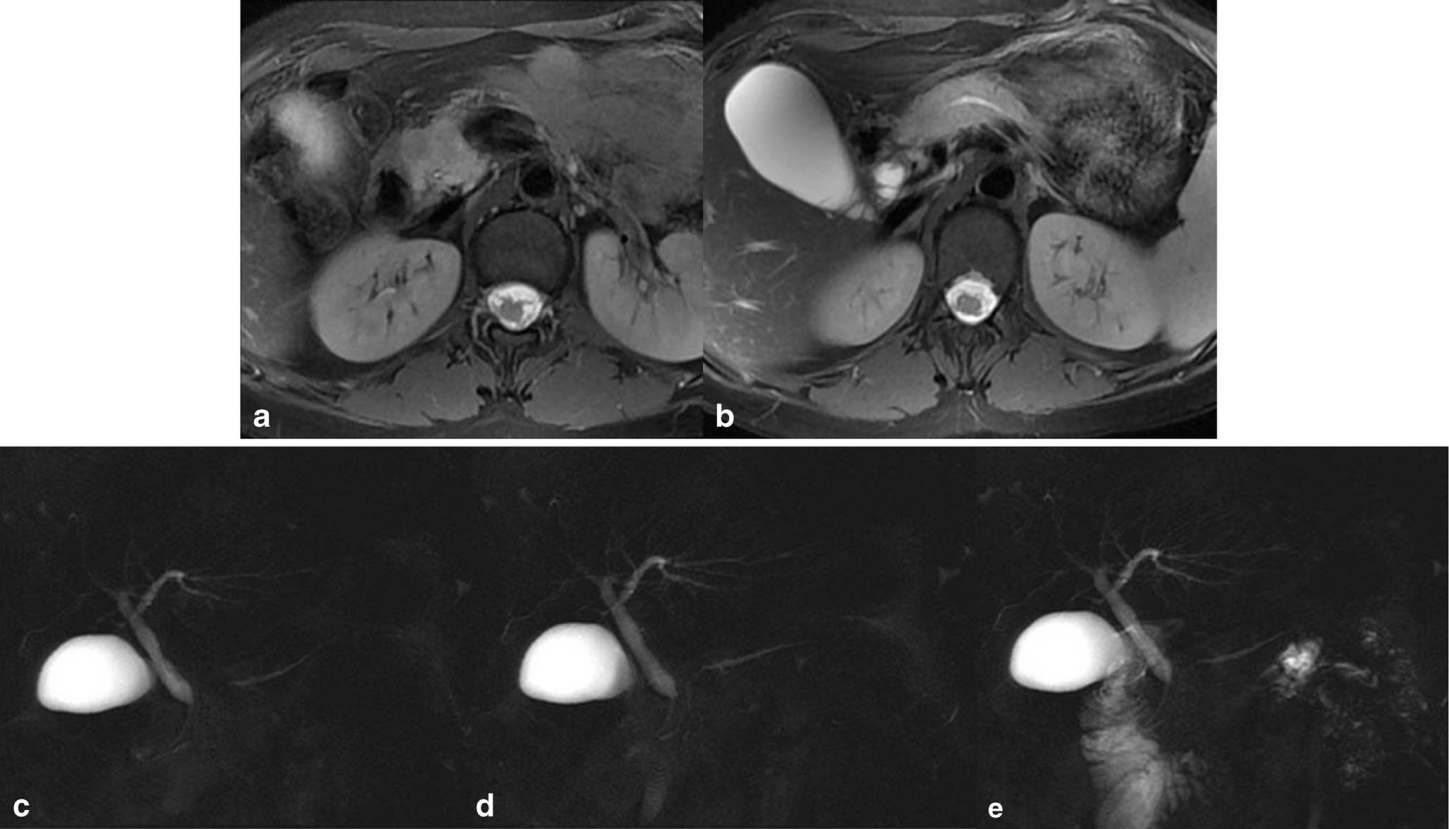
a–e Autoimmune pancreatitis. Axial T2-weighted images (a, b) show loss of the normal pancreatic lobulations at the level of the head and focal obliteration of the pancreatic duct, better demonstrated on conventional MRCP (c). After secretin administration (d, e) the stricture detected in basal phase is solved (duct-penetrating sign)
